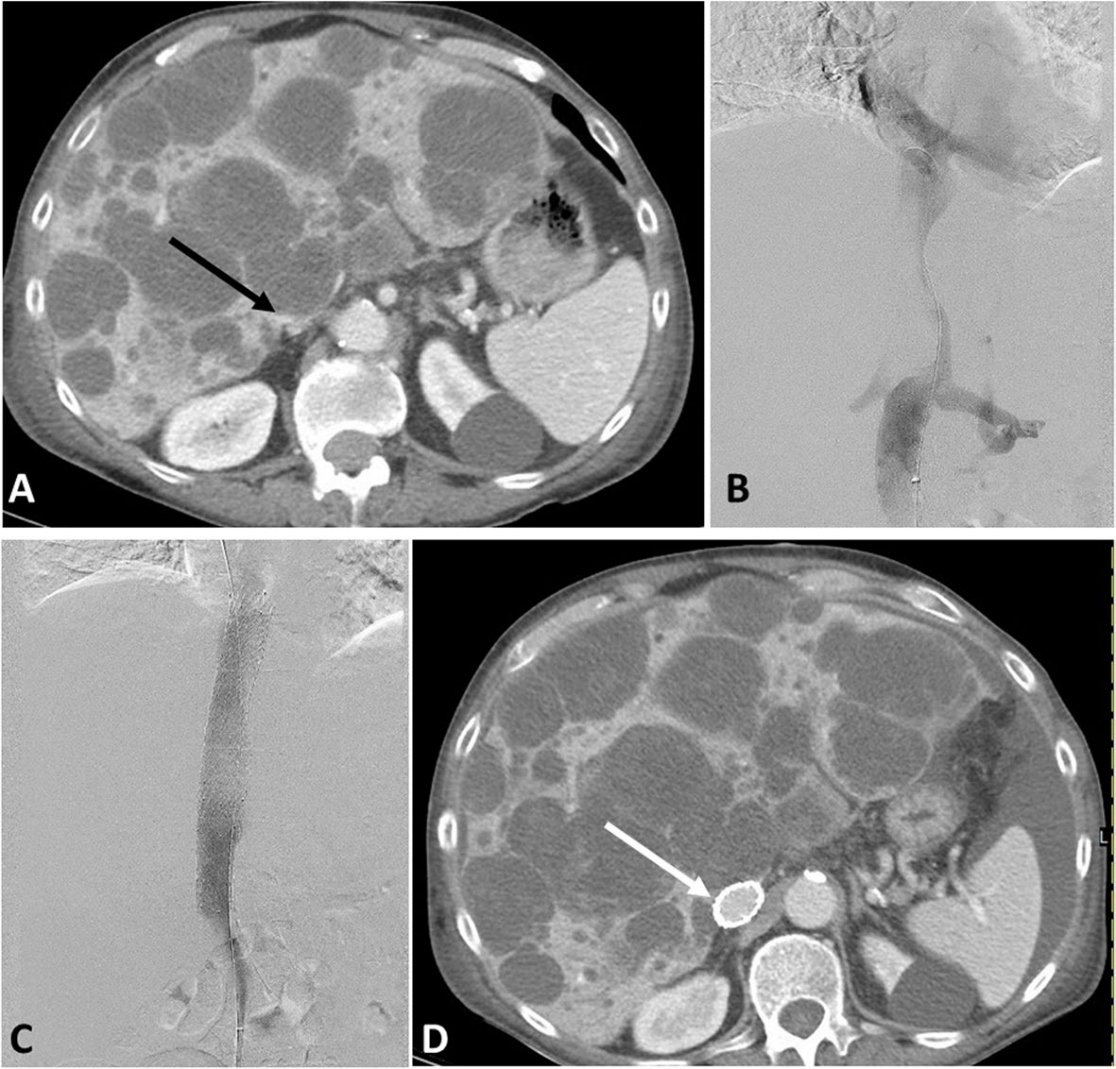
79 y male with metastatic chondrosarcoma presented with new onset of bilateral lower extremity swelling. A CT of the abdomen showing growing hepatic metastasis compressing the intrahepatic IVC (Black arrow). B Venography showing attenuation of the intrahepatic IVC. C Post stenting venography showing successful restoration of flow with disappearance of collaterals after placing 20 mm stent and post-stenting venoplasty using 20 mm balloon. D CT of the abdomen obtained one week later due to persistent abdominal pain showing patent stent and new bleeding into some of the hepatic lesions (not shown). Lower extremity swelling already improved
